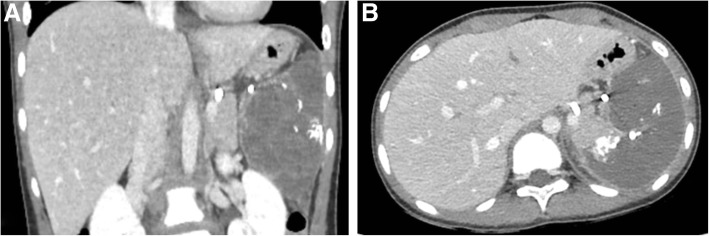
a and b Computed tomography on day 6 after embolization shows total infarction of the spleen. Embolic material at the distal splenic artery is also seen on the scans
